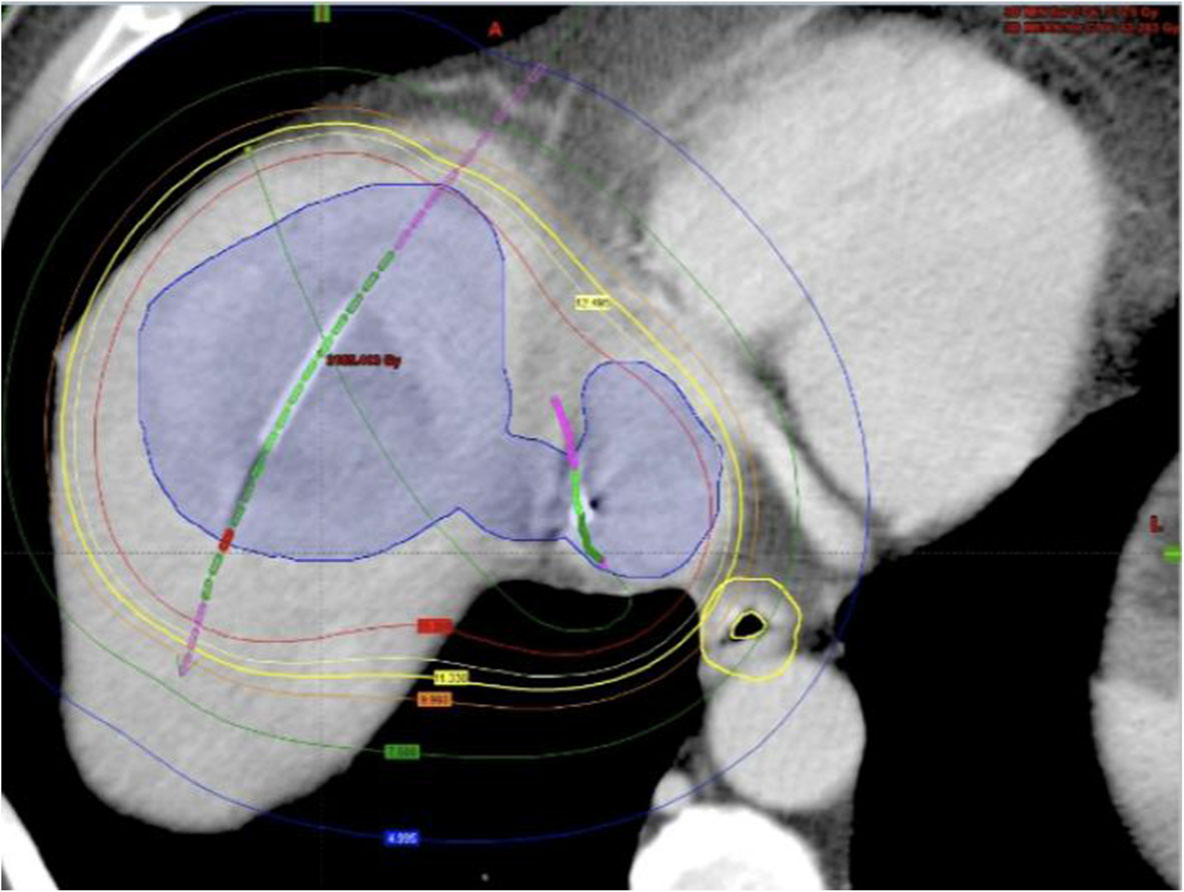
Peri-interventional 3D-irradiation plan. Contrast-enhanced planning computed tomography (CT) after CT-guided positioning of two afterloading catheters. Orange marker shows transcutaneous catheter 1, green marker shows transfemoral catheter 2. Visible tumour borders were defined as the clinical target volume (CTV) (blue line). Dose distribution was adjusted by 3D-treatment planning. The planned minimal enclosing dose was 15 Gy (blue line). Isodose irradiation lines surround the CTV
